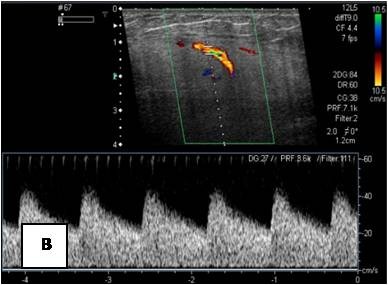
It contains prominent vessels with Doppler.
